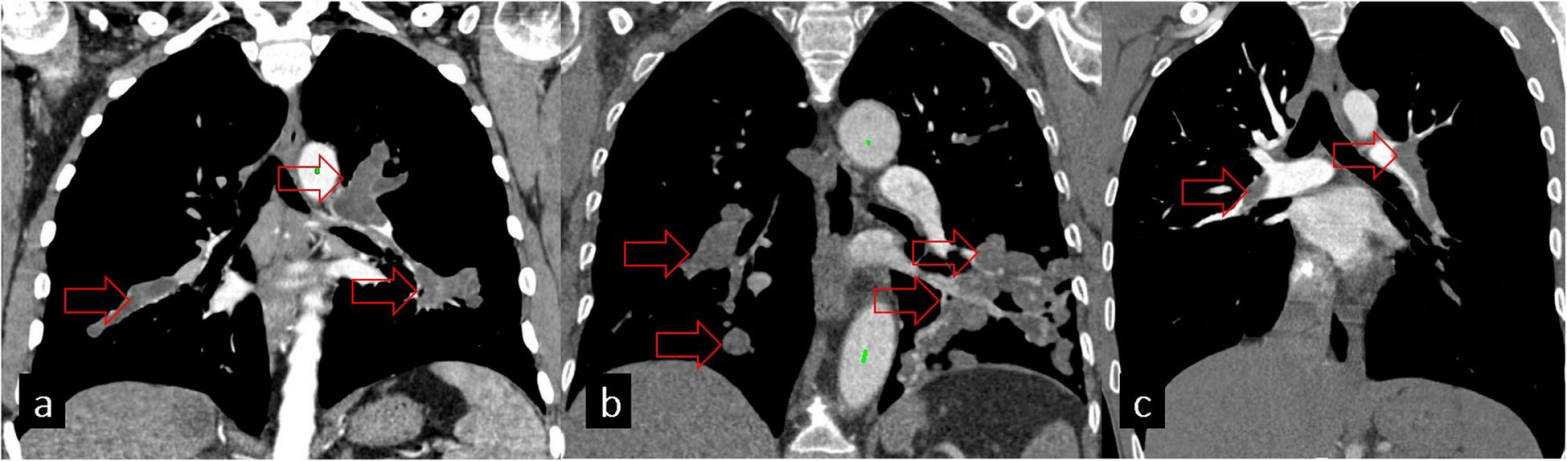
a Hydatid cysts of pulmonary arteries seen on the coronal chest CT image. b Sarcoma of the pulmonary artery shows vascular distension and fills the lumen like hydatid cysts of the pulmonary arteries. c Pulmonary thromboembolism as another differential diagnosis of hydatid cyst seen on the coronal chest CT image
